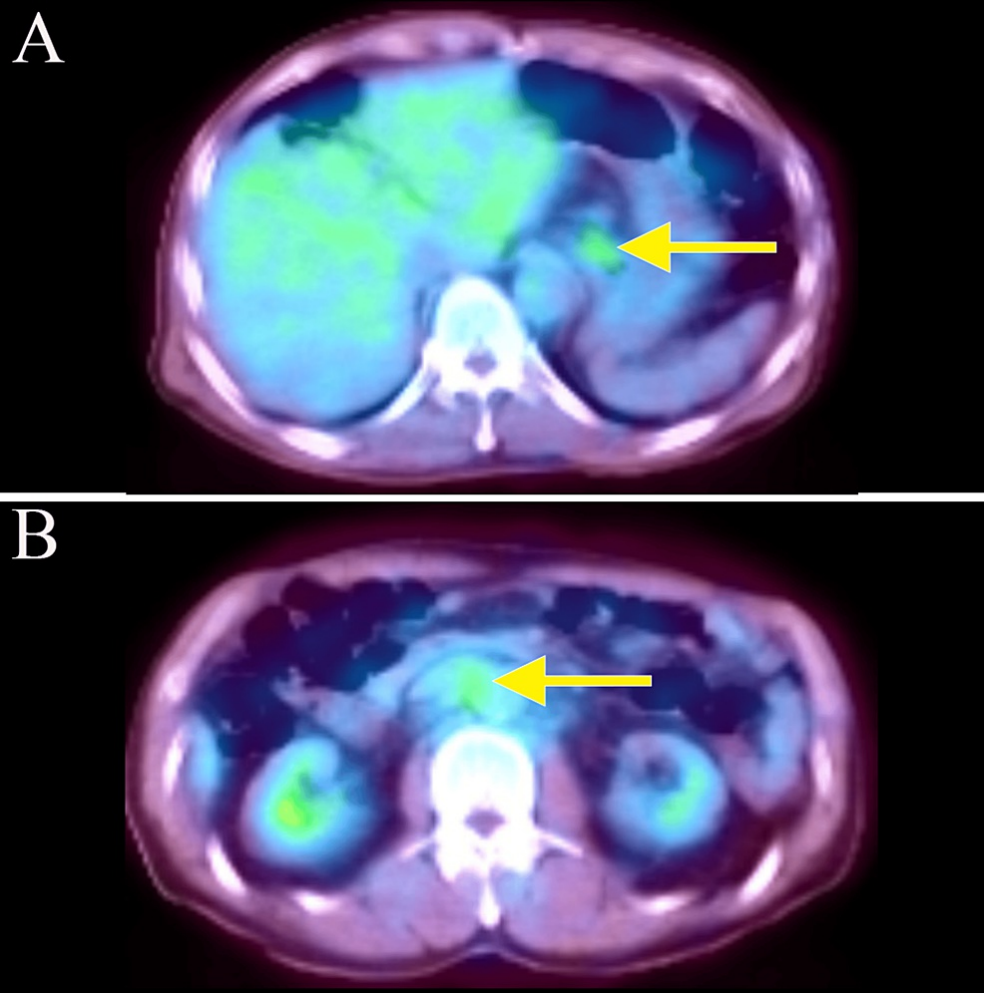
Positron emission tomography-computed tomography (A, B) Image taken before chemotherapy. Positron emission tomography (PET)-CT scans confirmed wall thickening at the hilum and multiple enlarged lymph nodes in the left supraclavicular fossa and around the aorta from the hilum to the upper abdomen. The wall thickening at the hilum had an SUVmax of 3.0; multiple enlarged lymph nodes around the aorta from the hilum to the upper abdomen had SUVmax values ranging from 2.5 to 3.0.
